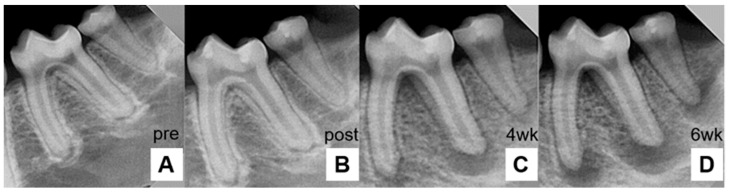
Time-dependent radiographic changes in the periapical lesion. (A) Pre-experiment, (B) post-bacterial inoculation, (C) 4 weeks after inoculation, and (D) 6 weeks after inoculation. Bacteria were injected into a healthy periapical environment. After 4 weeks, a distinct periapical lesion appeared. After 6 weeks, the lesion had enlarged markedly.
